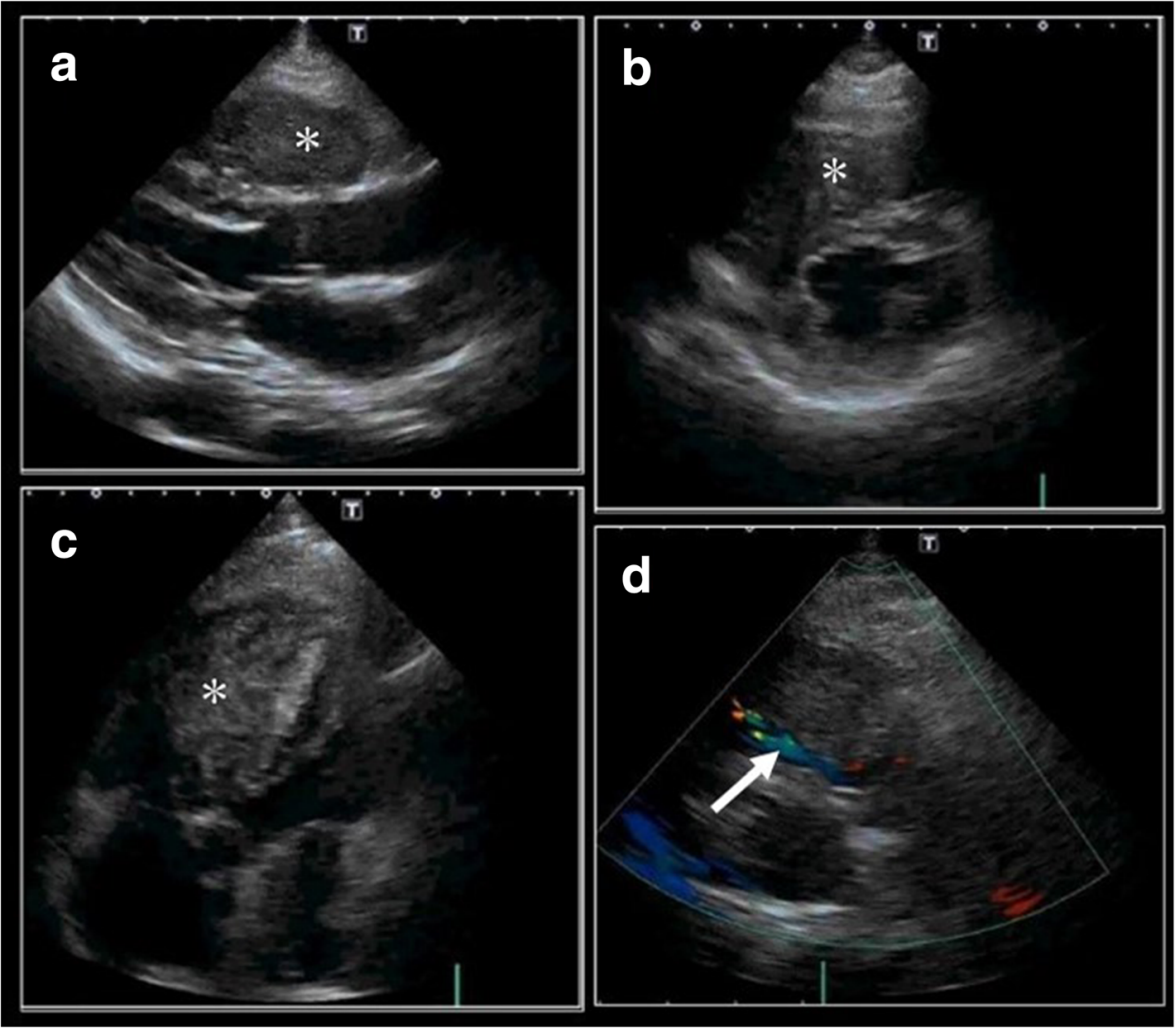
Echocardiograms. a–c Echocardiograms revealing a huge mass occupying the right ventricle (*). There were no masses in the right atrium. The patient’s right ventricular pressure was elevated, and the interventricular septum was flattened. d Color Doppler echocardiogram showing a disturbance in right ventricular outflow (*white arrow*)
